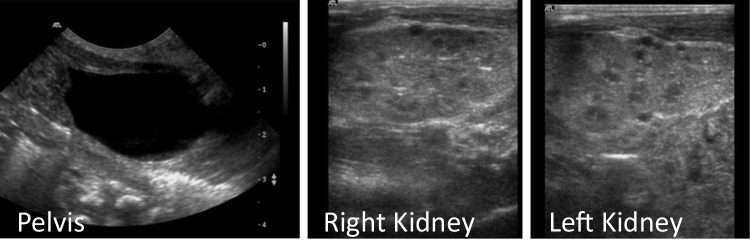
Kidney ultrasound of Twin A during the neonatal period showing bilateral kidney enlargement, increased echogenicity, and multiple cysts
